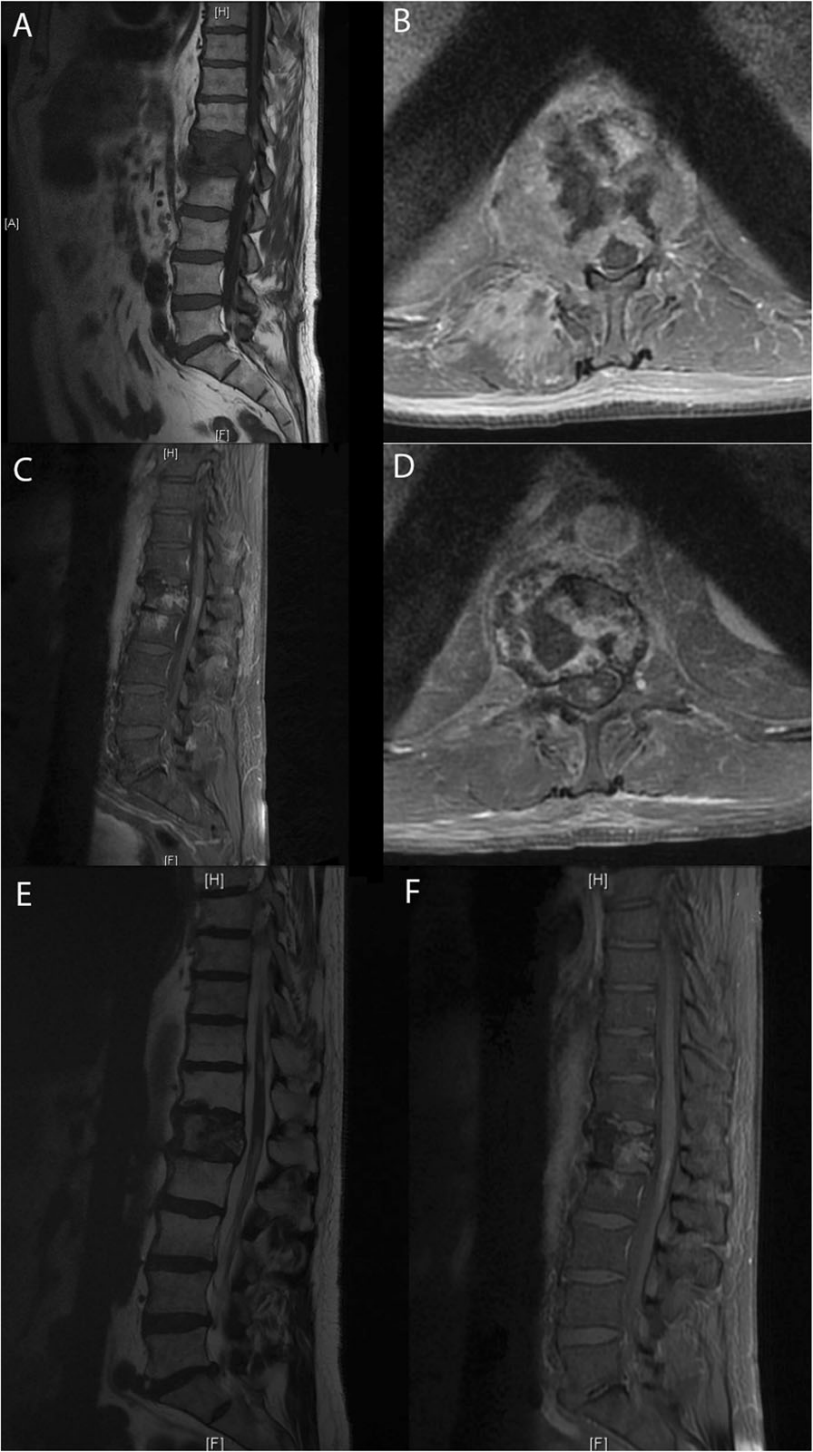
The radiological evolution of myelitis. a and b MRI performed at the time of epiduritis diagnosis, sagittal T1-weighted spin-echo and axial fat-suppressed T1 after gadolinium injection show osseous metastasis of L1 with epiduritis (but no enhancement of the spinal cord). c and d MRI after the first signs of myelitis, sagittal and axial fat-suppressed T1 after gadolinium injection show abnormal enhancement of the conus medullaris, and regression of osseous involvement and epiduritis. e MRI at 1 month after the discontinuation of immunotherapy, sagittal T2-weighted spin-echo shows hyperintensity of the conus medullaris. f MRI at 3.5 months, sagittal fat-suppressed T1 after gadolinium injection shows the persistence of conus medullaris enhancement
